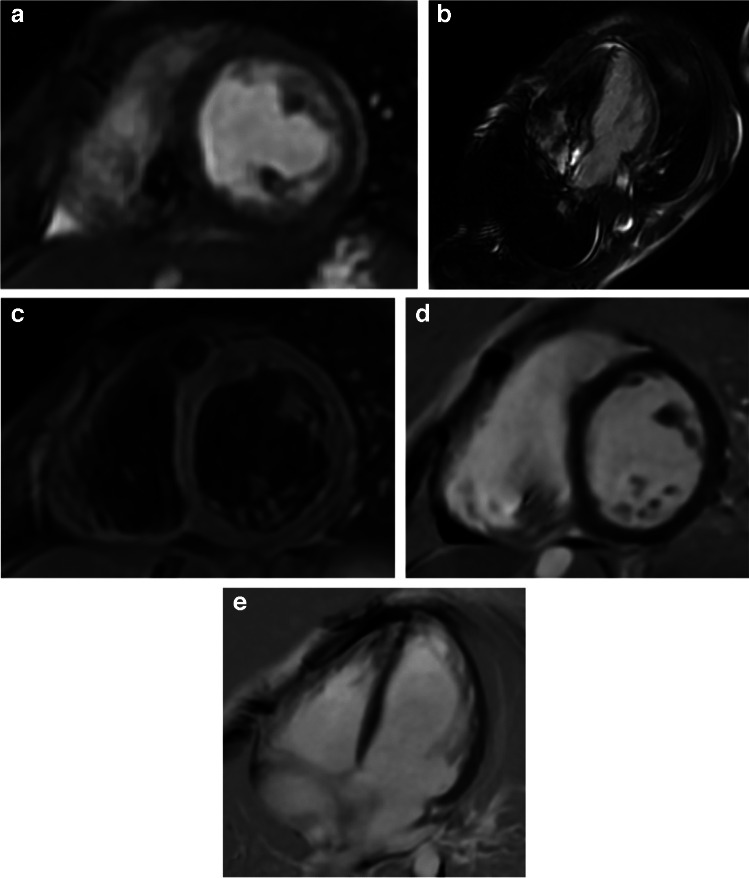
A 6-year-old girl with high-degree atrioventricular (AV) heart block. a Short-axis, unenhanced, balanced steady-state free precession (bSSFP) cardiac MR image shows acceptable image quality for evaluating cardiac motion and myocardial structure with minimal artifact. b Four-chamber unenhanced bSSFP cardiac MR image demonstrates susceptibility artifact in the right chest wall related to the externalized pacemaker generator. Susceptibility artifacts are also observed in the right cardiac chambers due to the pacing lead. These artifacts affected <5% of the imaging field and did not impact functional analysis. c Short-axis unenhanced triple inversion recovery T2-weighted cardiac MR image shows no myocardial edema and no significant artifact. d Short-axis fast gradient echo (FGRE) late gadolinium enhancement (LGE) cardiac MR image shows no abnormal myocardial enhancement. e Four-chamber FGRE late gadolinium enhancement (LGE) cardiac MR image shows no abnormal myocardial enhancement
